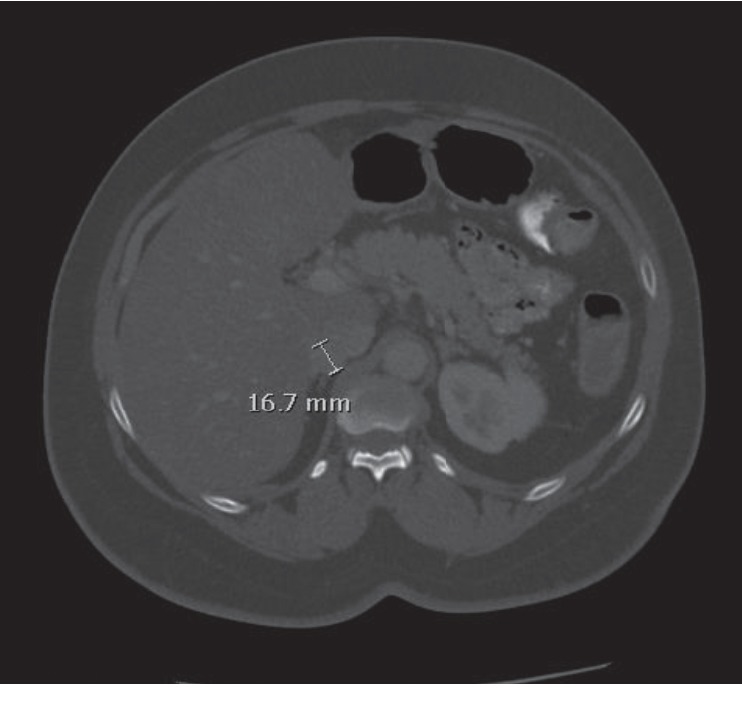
Follow-up CT noting an increase in size of the right adrenal nodule (1.7 cm) with 60 Hounsfield units of attenuation (right).
